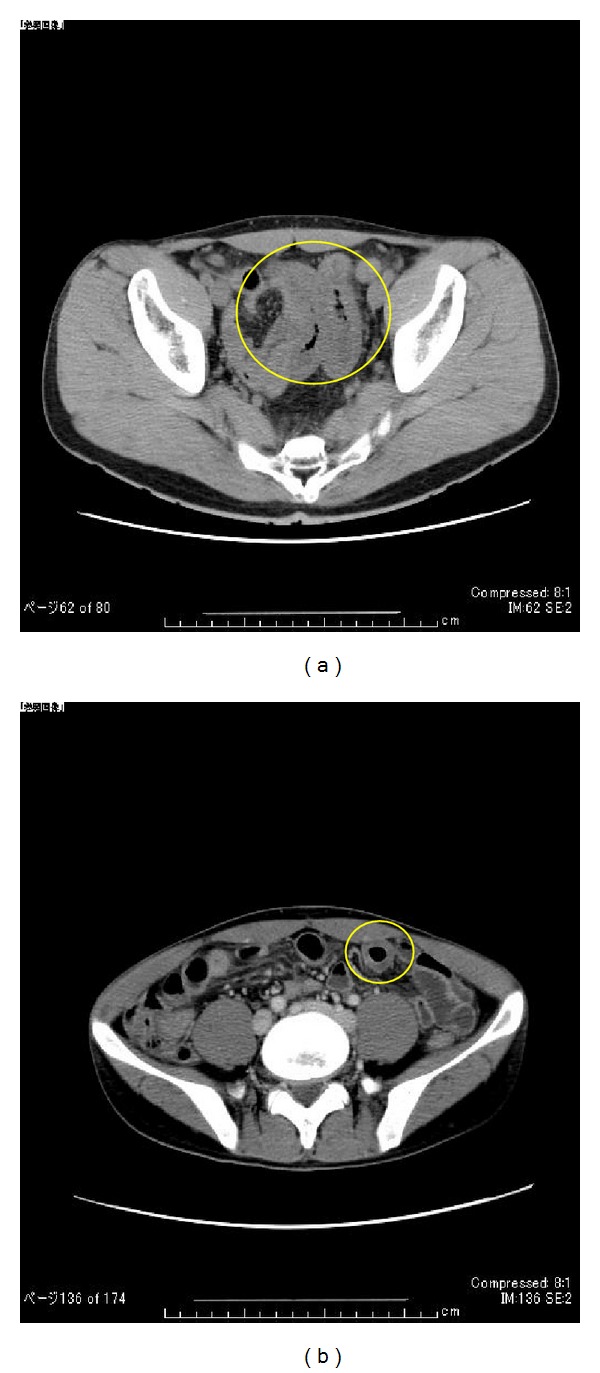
(a) CT scan in December 2009; thickening of small intestine wall is circled. (b) Contrast-enhanced CT scan in February 2010; edema-like contrast effect of intestinal wall thickening is circled.
